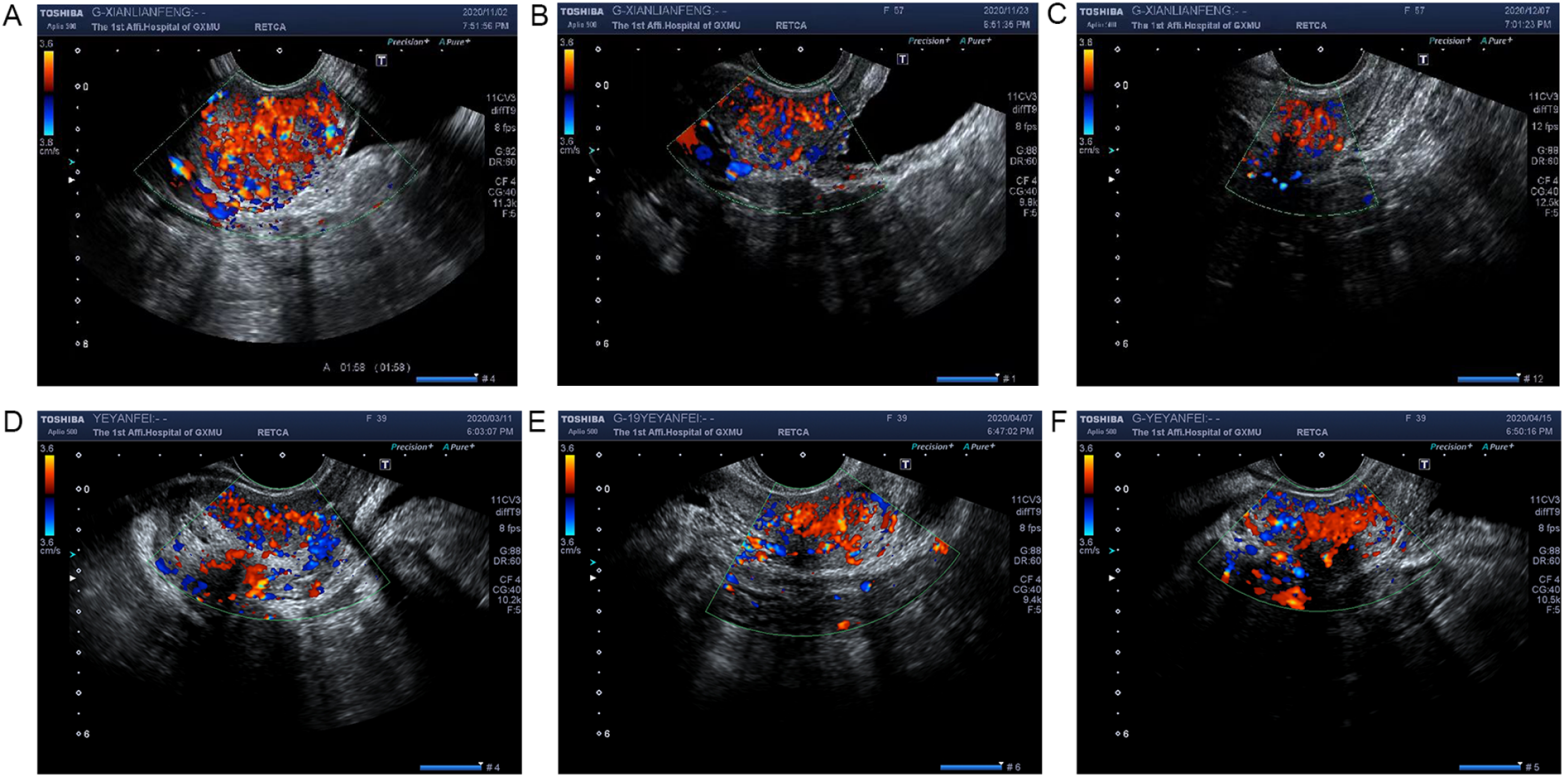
Color Doppler ultrasonography imaging of cervical cancer. (A–C) Representative color Doppler ultrasonography images of cervical cancer before (A), during (B), and after (C) radiotherapy in patients of the CRT+E group, demonstrating changes in tumor vascularity over the course of treatment. (D–F) Representative color Doppler ultrasonography images of cervical cancer before (D), during (E), and after (F) radiotherapy in patients of the CRT group, showing differences in vascular morphology and perfusion compared to the CRT+E group.
